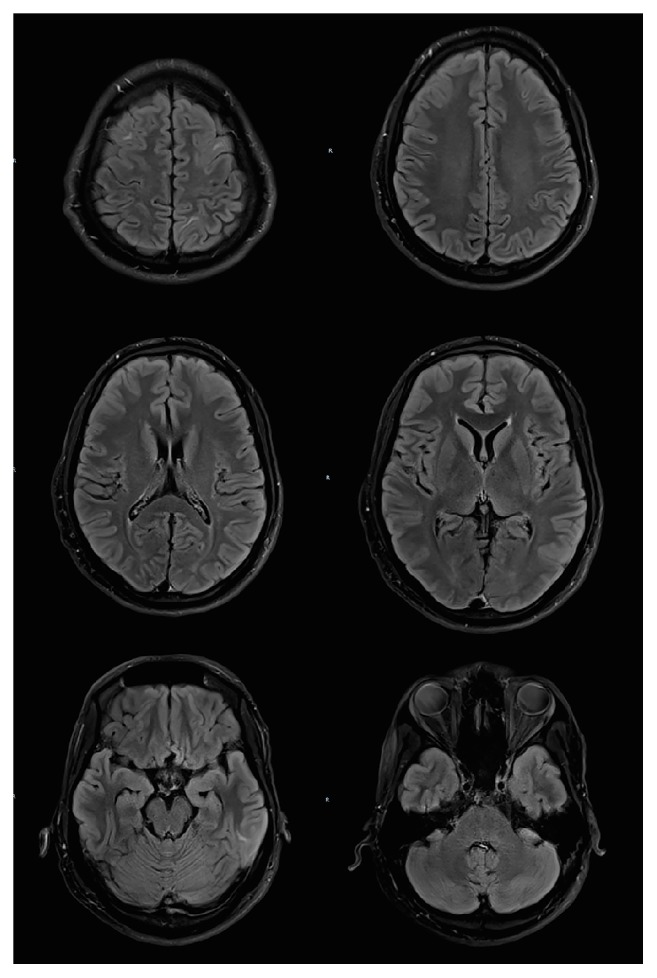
T2 FLAIR sequence. MRI brain done on Day 14 of heat stroke showed near complete resolution of the abnormal cortical and subcortical signal intensity and swelling, compatible with diagnosis of PRES.
